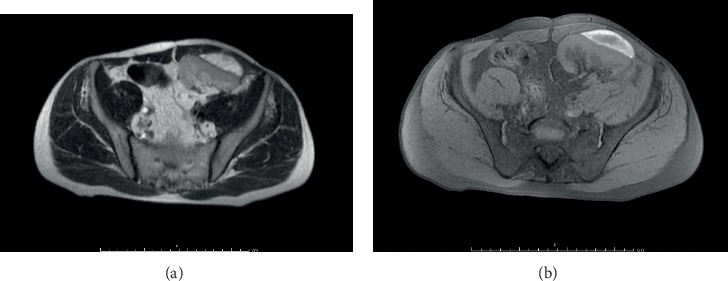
Contrast-enhanced magnetic resonance imaging at day 9 showing the dimensional reduction of the subcapsular hematoma in the transplanted kidney and the absence of fluids in the perirenal spaces. (a) Axial T2-weighted. (b) Axial T1-weighted.
